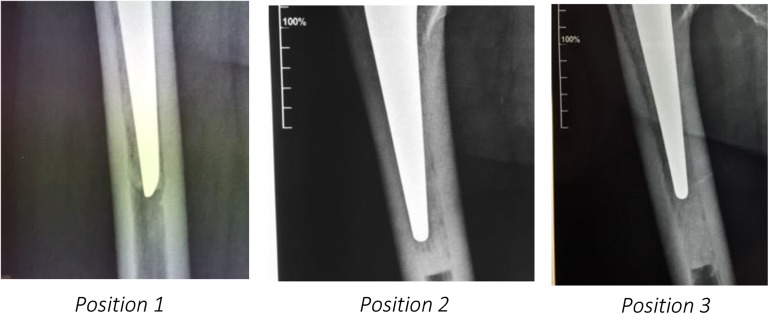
X-ray evaluation of APCR position related to the tip of the stem.
